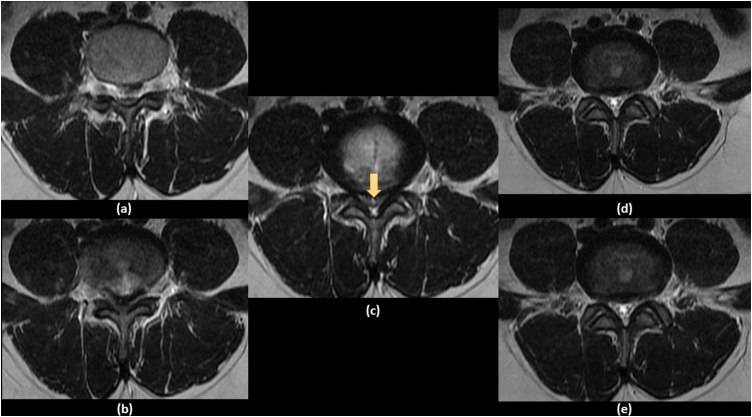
Sequential axial T2 weighted MRI images showing huge central L4 L5 disc herniation: (a) two cuts above L4 L5 disc, (b) one cut above L4 L5 disc, (c) cut at L4 L5 Disc, (d) one cut below L4 L5 disc and (d) two cuts below L4 L5 disc, (e) two cuts above L4 L5 disc.
